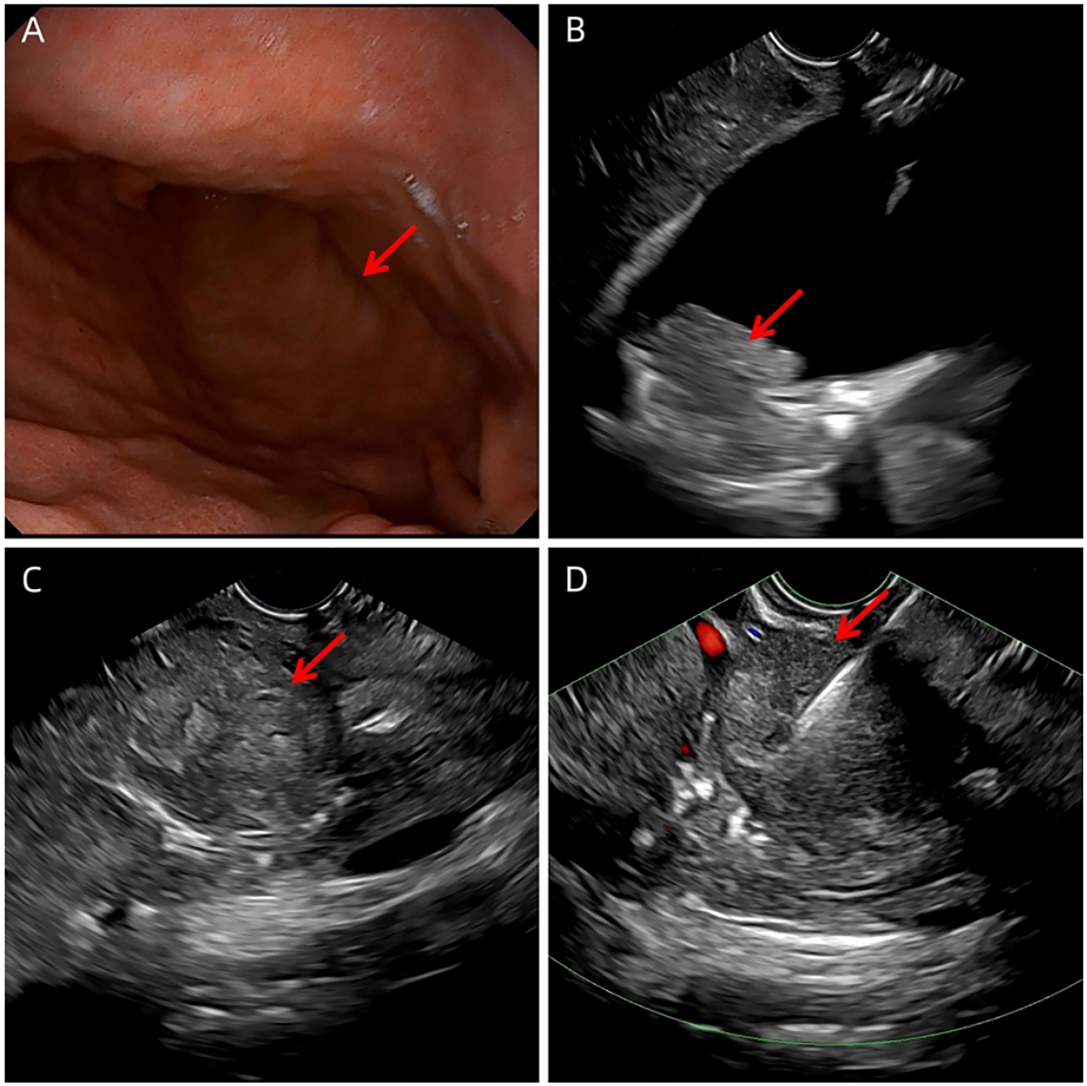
Radiological and endoscopic evaluation of the patient. (A) Endoscopic image showing a submucosal protrusion in the stomach, caused by an enlarged retroperitoneal lymph node protruding into the gastric lumen. (B) Abdominal ultrasound image demonstrating a solid, dumbbell-shaped mass located at the neck of the gallbladder (arrows),indicating invasion through the gallbladder wall. (C) Ultrasound image showing the enlarged retroperitoneal lymph node. (D) Ultrasound image guiding fine-needle aspiration (FNA).
